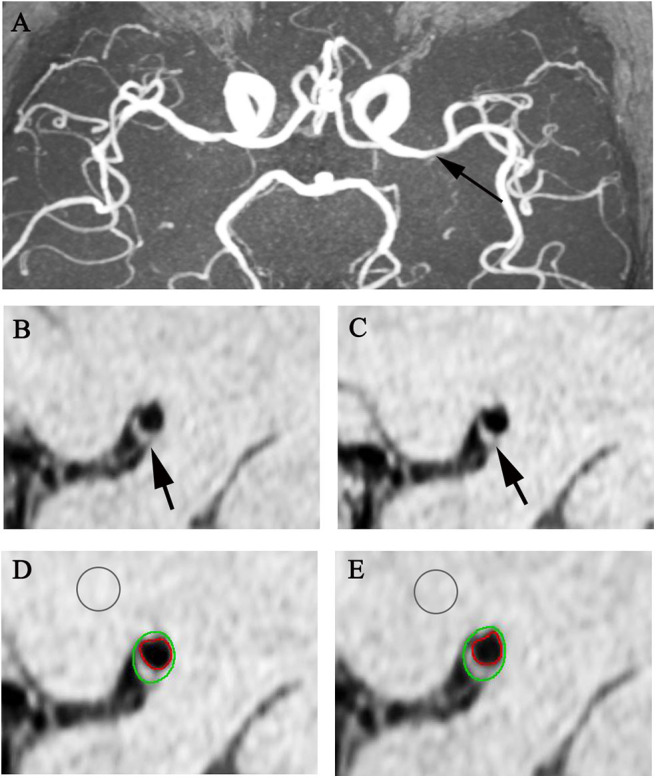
Representative MR images showing enhancement index measurement. A 65-year-old man with acute stroke showed severe left MCA stenosis on MRA (A arrow). After matching pre- (B,D) and post-contrast T1w images (C,E) at the most stenotic site, the plaque enhancement index of the plaque (arrows) is 2.82%.
